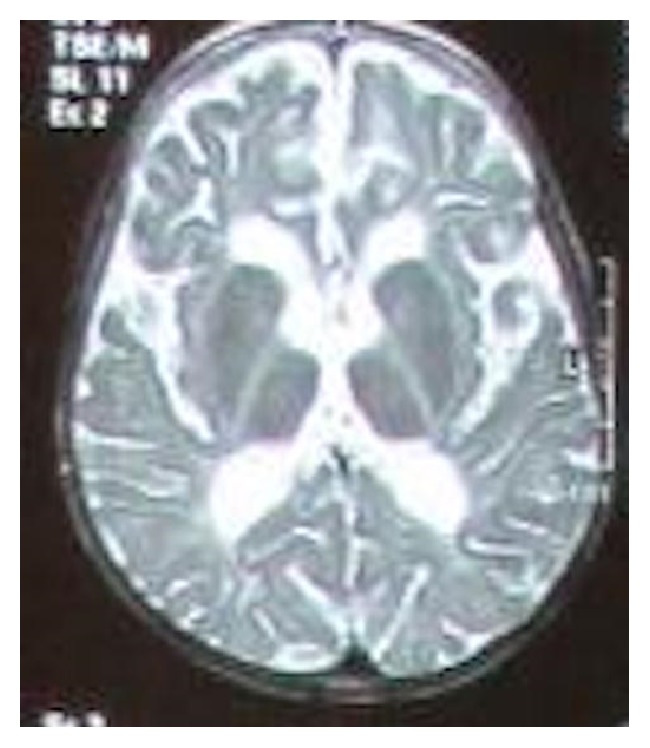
Brain MRI of the patient shows cerebral atrophy. Note hypomyelination and leukodystrophy.
